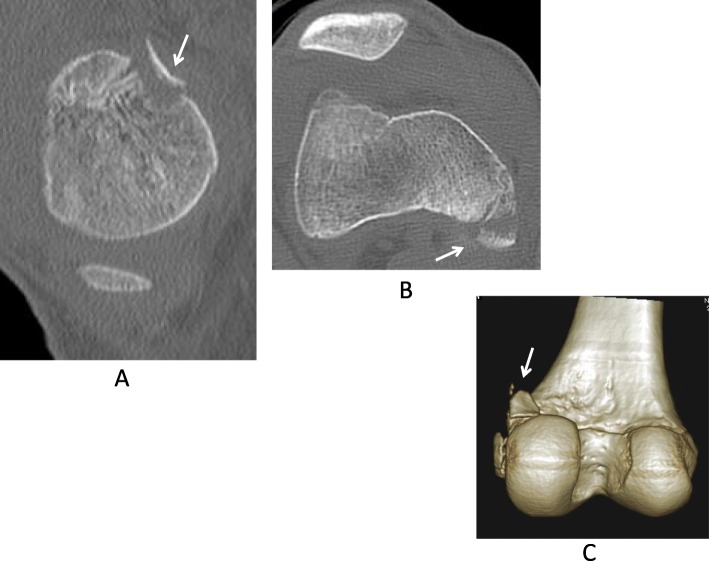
a and b and c Computed tomography of the injured knee. An avulsion fracture of the medial head of the gastrocnemius muscle in the sagittal plane (*arrow*, a), and in the axial plane (*arrow*, b), and in the three-dimensional computed tomography (*arrow*, c)
